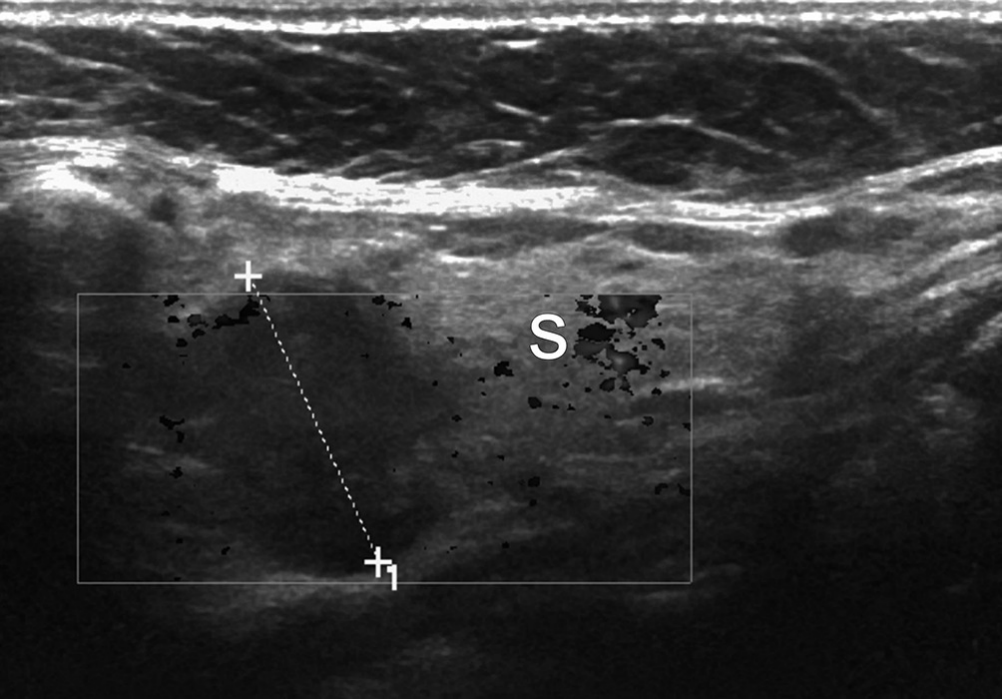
Colour Doppler ultrasound image corresponding to the FDG-avid region on PET-CT. Ill-defined hypoechoic mass within the medial left submandibular gland (calipers), avascular and heterogeneous features those of malignancy though non-specific in differentiating primary from secondary neoplasm.
